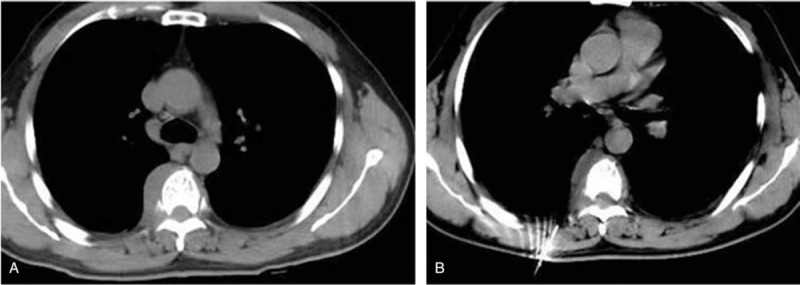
(A and B) Enhanced CT scan and bone marrow biopsy of paravertebral tissue guided by CT.
